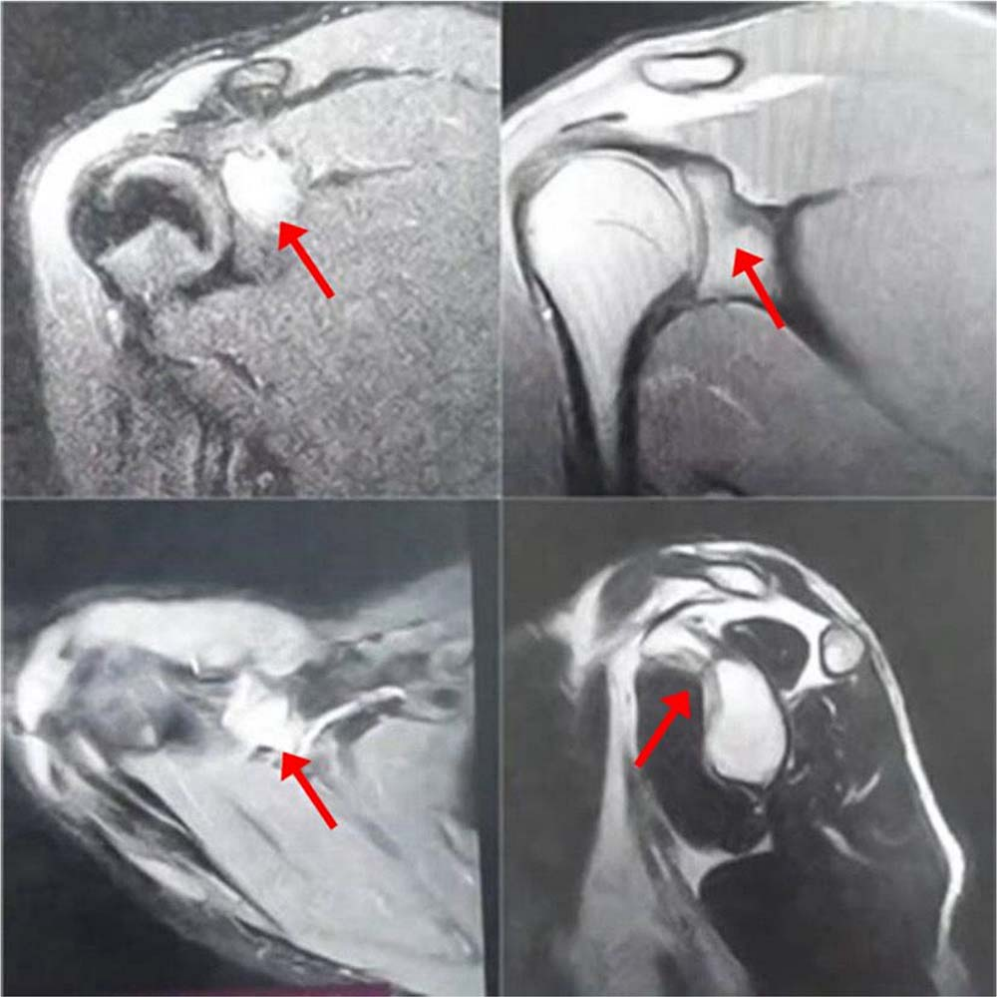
MRI without contrast of the left shoulder show a cortical and subcortical bony defect at the glenoid basecoracoid junction with hyperintense lesion measuring 8 × 7 mm (indicated by arrow), also intense bone marrow edema at the coracoid processes and the glenoid base-coracoid with surrounding fluid and edema extending to the coracoid-humeral space was seen.
